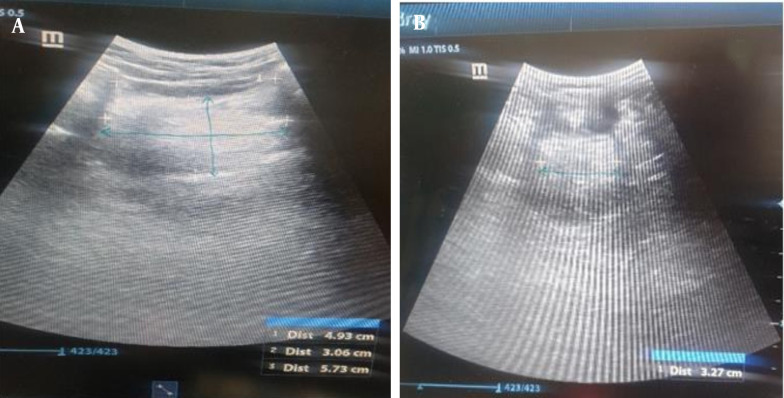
A, Midsagittal cross-sectional area of the tongue and B, the width of the tongue obtained from transverse sonograms (blue arrow).
